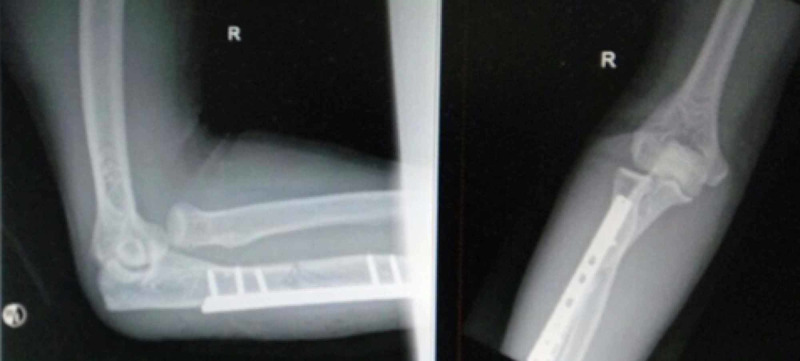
Radiograph showing irreducible radial head dislocation after fixation of ulna fracture (AP and lateral view).
